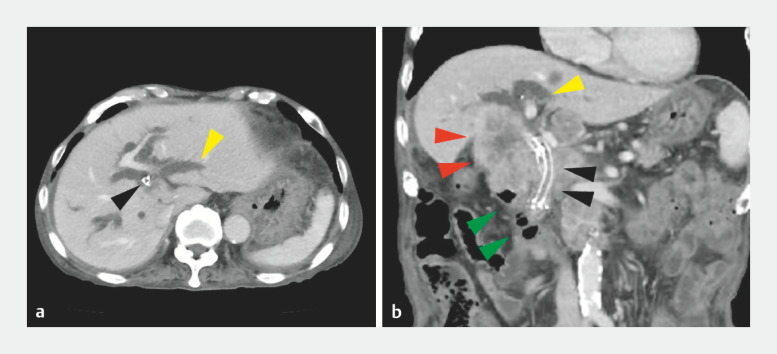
Contrast-enhanced computed tomography (CT) showing dilatation of the bilateral intrahepatic bile duct (B2 branch, yellow arrowheads), a huge mass formed by biliary cancer and metastatic lymph node (red arrowheads), biliary covered metallic stents placed side by side (black arrowheads), and stenosis of the duodenum due to infiltration of the tumor (green arrowheads).
